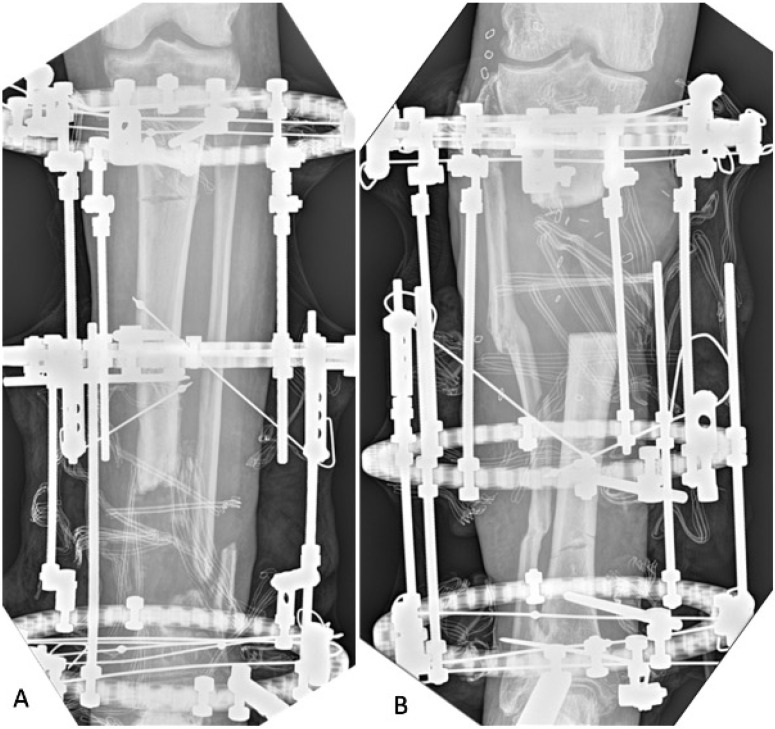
X-rays of two different patients treated by resection of the non-union site and anterograde (A) or retrograde (B) bone transport.
